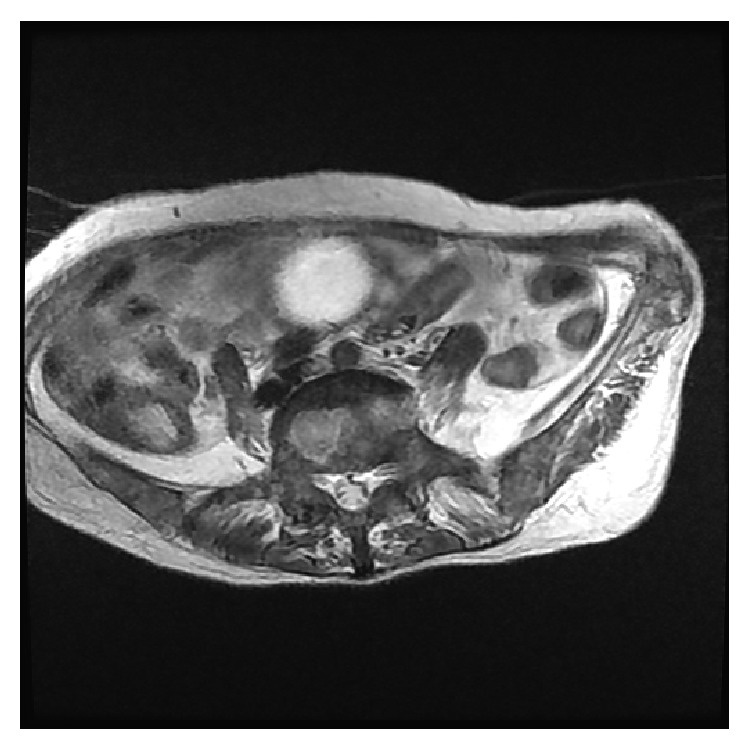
T1-weighted MRI revealed low-intensity lesion occupying lumbar spine and iliac bone diffusely.
